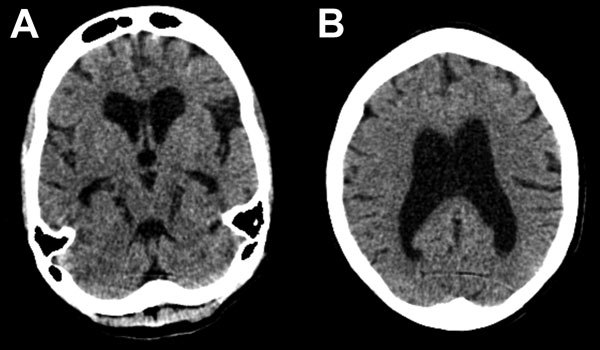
Representative axial cuts from noncontrast head computed tomography scan imaging of a 30-year-old woman with encephalitis resulting from Ebola virus infection, Sierra Leone. Images show global atrophy in keeping with nonobstructive ventriculomegaly and no periventricular low attenuation: A) subcortical atrophy; B) cortical atrophy. There was no evidence of hydrocephalus, previous stroke, or intracranial hemorrhage. A cavum septum pellucidum was noted in other images.
